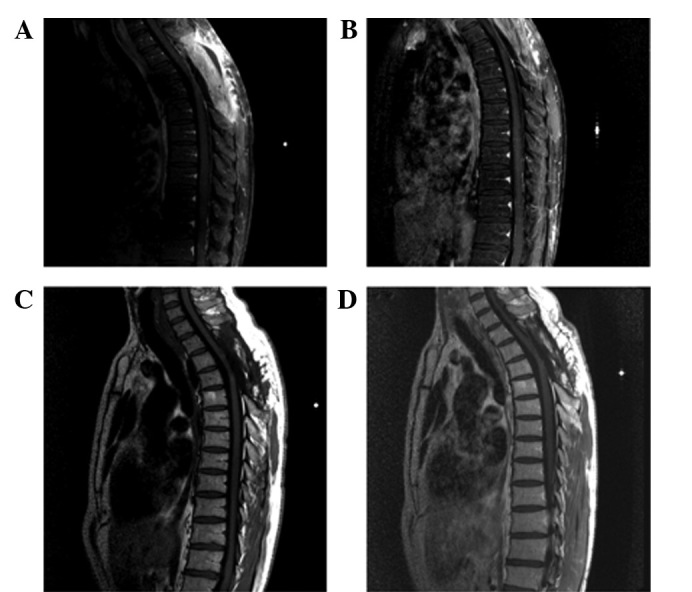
MRI images following tumour incision (T1-contrast). No lesion was observed in follow-up MRI at (A) 3 months, (B) 6 months, (C) 12 months and (D) 18 months after surgery. There was a small myelomalacia in the spinal cord.
